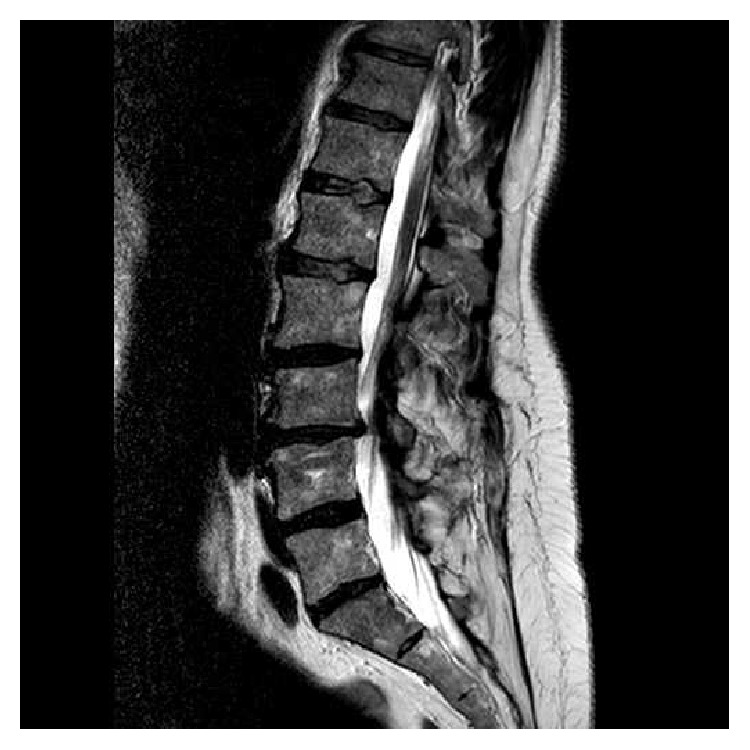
Sagittal T1-weighted MR imaging of the lumbar spine of a 55-yo patient with a previous rigid fixation on L4L5S1 level shows adjacent segment disease at the L3L4 level.
